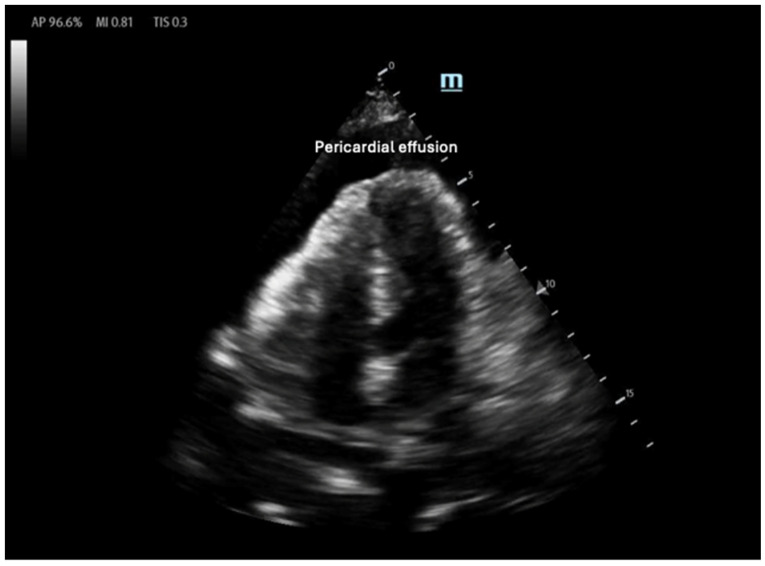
Anechoic pericardial effusion in apical 4-chamber view.
